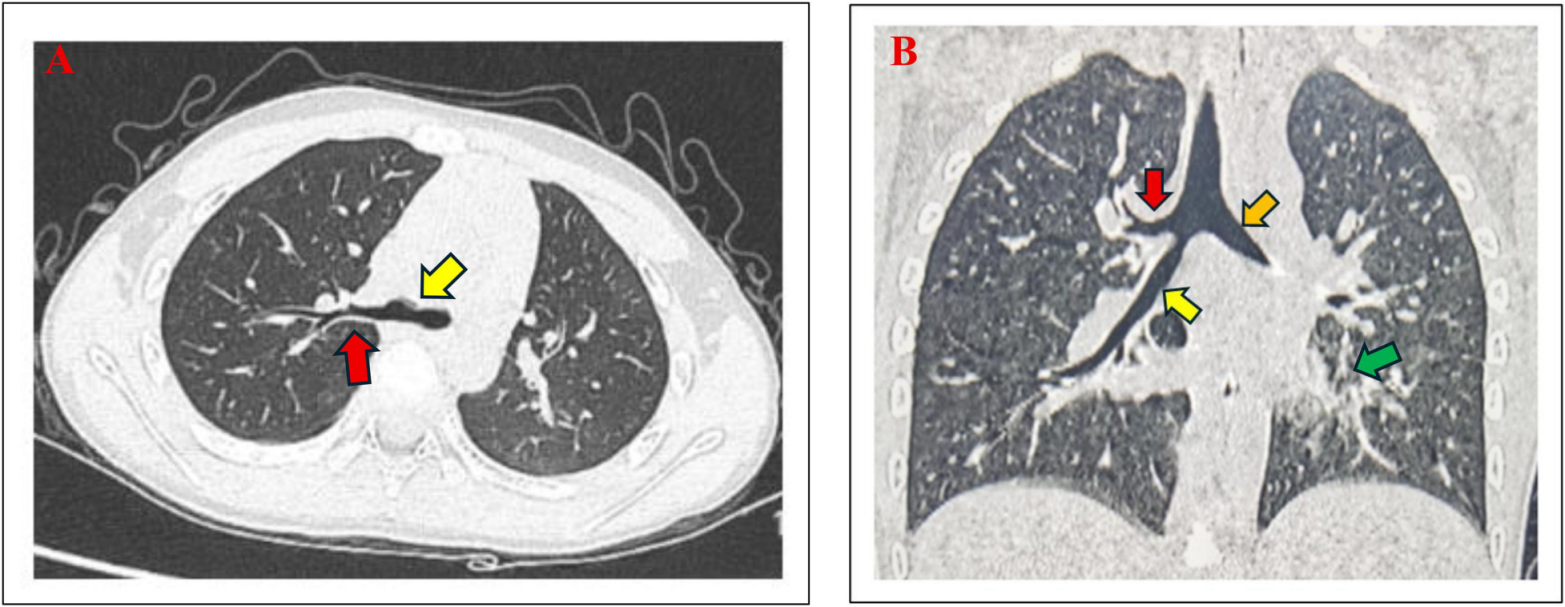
(A,B) Chest computed tomography (CT) findings. (A) Axial view (lung window) showed revealing tracheal bronchus (red arrow) over the right-sided distal tracheal (yellow arrow) above the carina. The right upper lobe bronchus was absent at its typical branching site. (B) Coronal view demonstrated the tracheal bronchus (red arrow) arising superior to the carina, along with the right main bronchus (yellow arrow) and left main bronchus (orange arrow). Patchy consolidation and mucus impaction were evident in the left lower lobe (green arrow).
